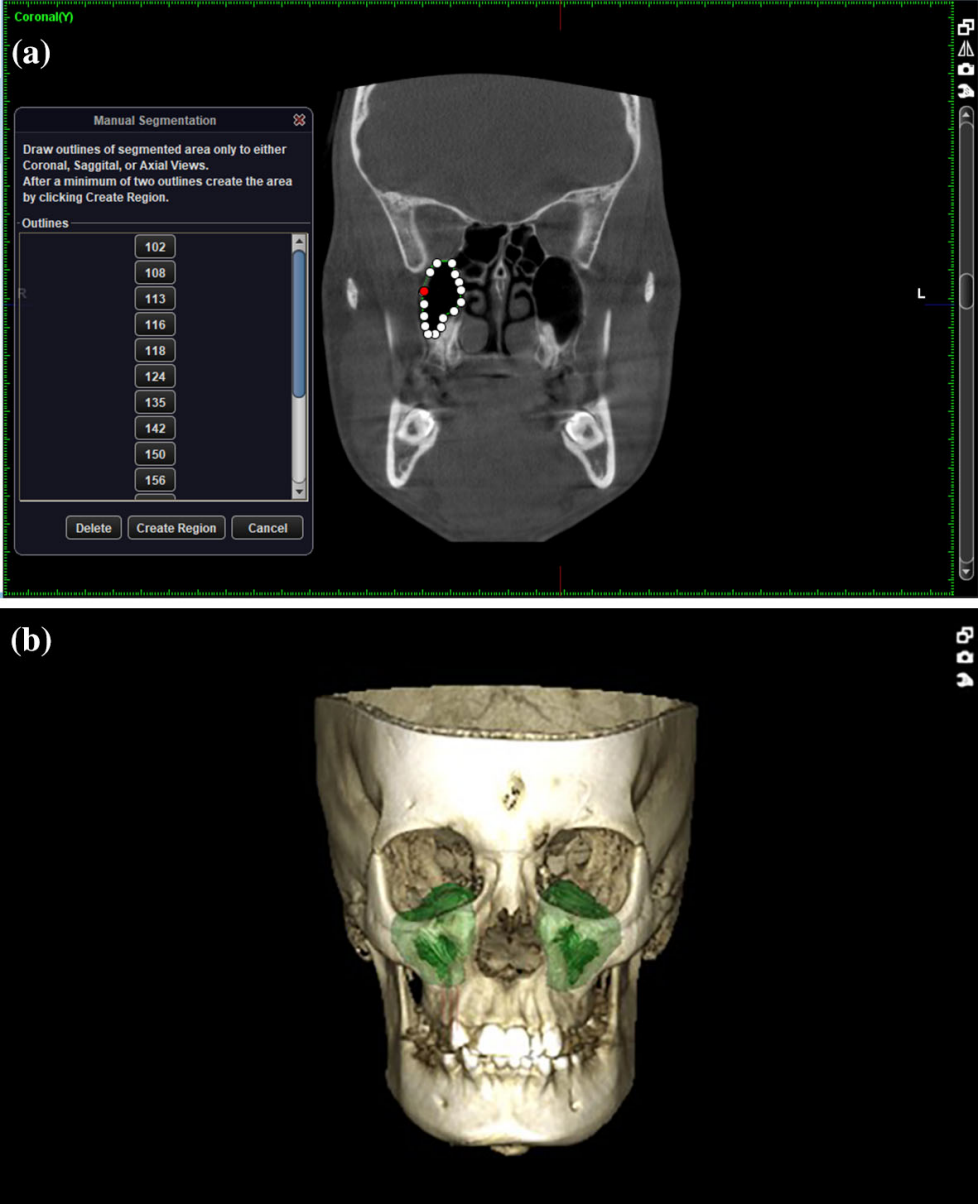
a - Volume of the maxillary sinus (marking the boundary for each CBCT slice). b - Constructed 3D view of sinus.
